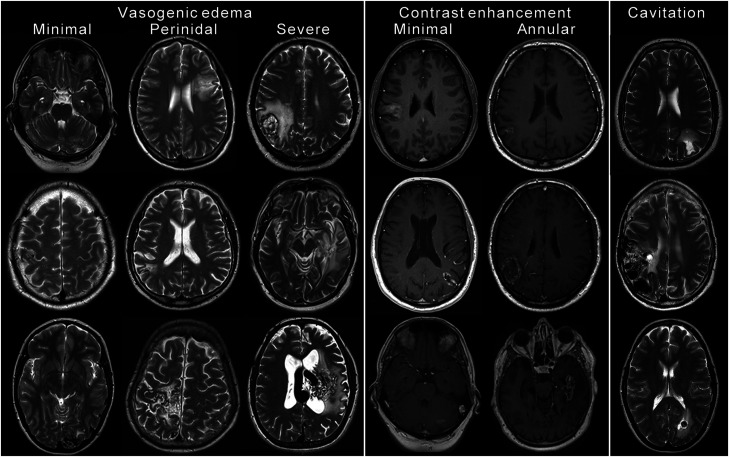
Example images from different patients demonstrating radiation-induced MRI findings after proton-treated AVMs according to our classification; vasogenic edema (minimal, perinidal, or severe), contrast enhancement (minimal or annular), and cavitation. MRI: Magnetic resonance imaging; AVMs: Arteriovenous malformations.
